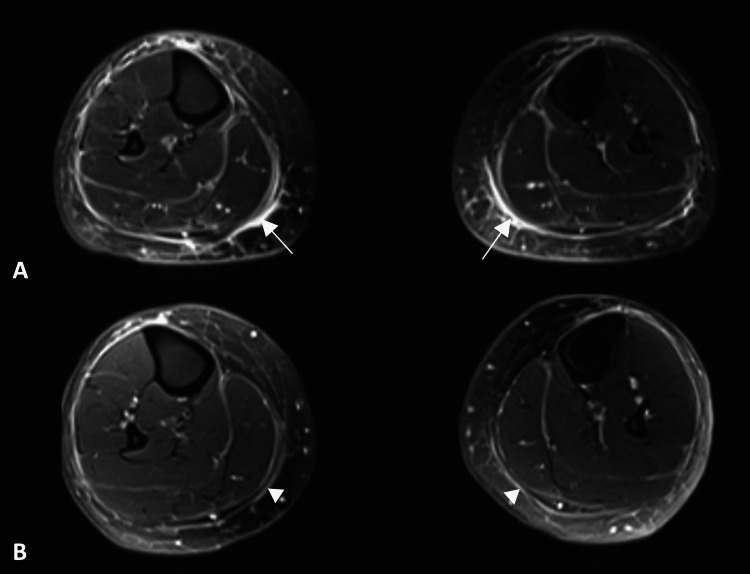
(A) Axial T2-weighted fat-suppressed MRI image demonstrating thickening of deep peripheral fasciae (arrows) of both lower legs, visualized as high signal intensity in the affected areas. (B) A repeat MRI was performed after three months, showing a clear reduction in the fascial thickening in the same region (arrowheads).
